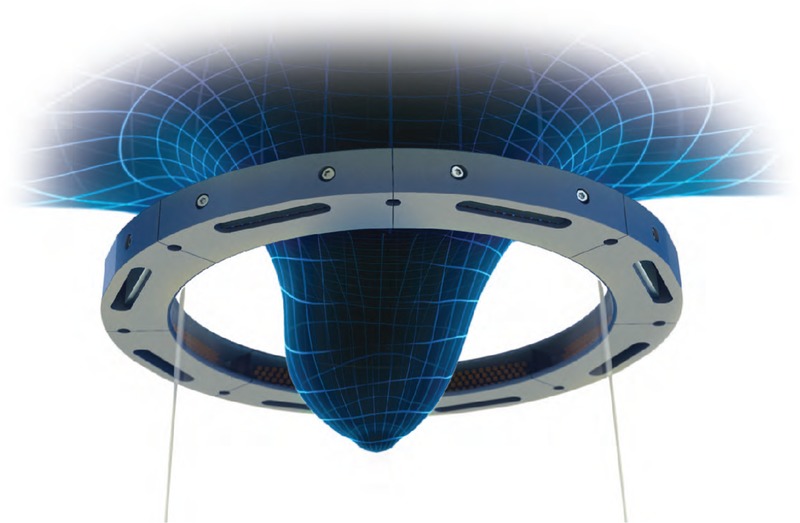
The SoftVue (Delphinus Medical Technologies) ultrasound ring array surrounds the breast and acquires images coronally moving away from the chest wall in 2.5-mm increments.
